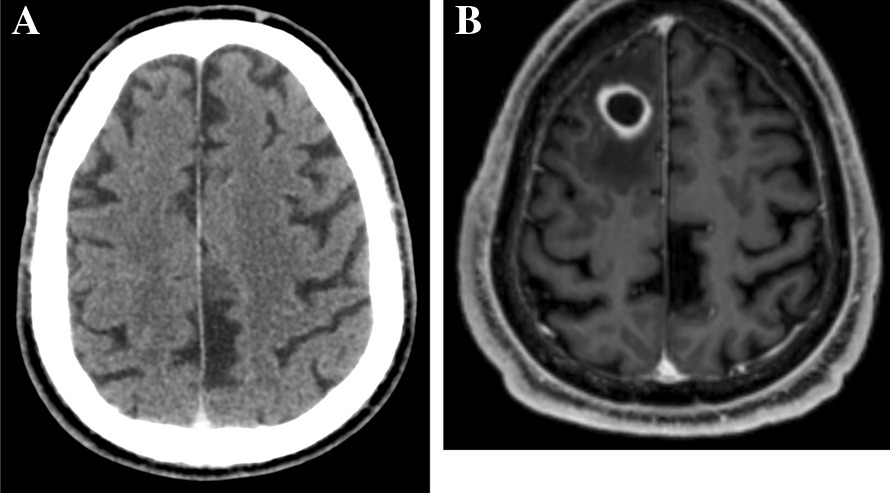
Brain CT before pembrolizumab start, and brain MRI at the time of cerebral nocardiosis diagnosis. A Brain CT before pembrolizumab start (no abnormality). B T1-weighted gadolinium-enhanced brain MRI at the time of cerebral nocardiosis diagnosis: 18 mm right frontal lobe round-shaped lesion with perilesional edema and wall enhancement
